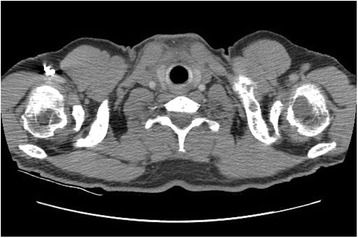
Thoracic computed tomography showing lytic lesions of the left clavicle and left humeral head and multiple lacunar foci
